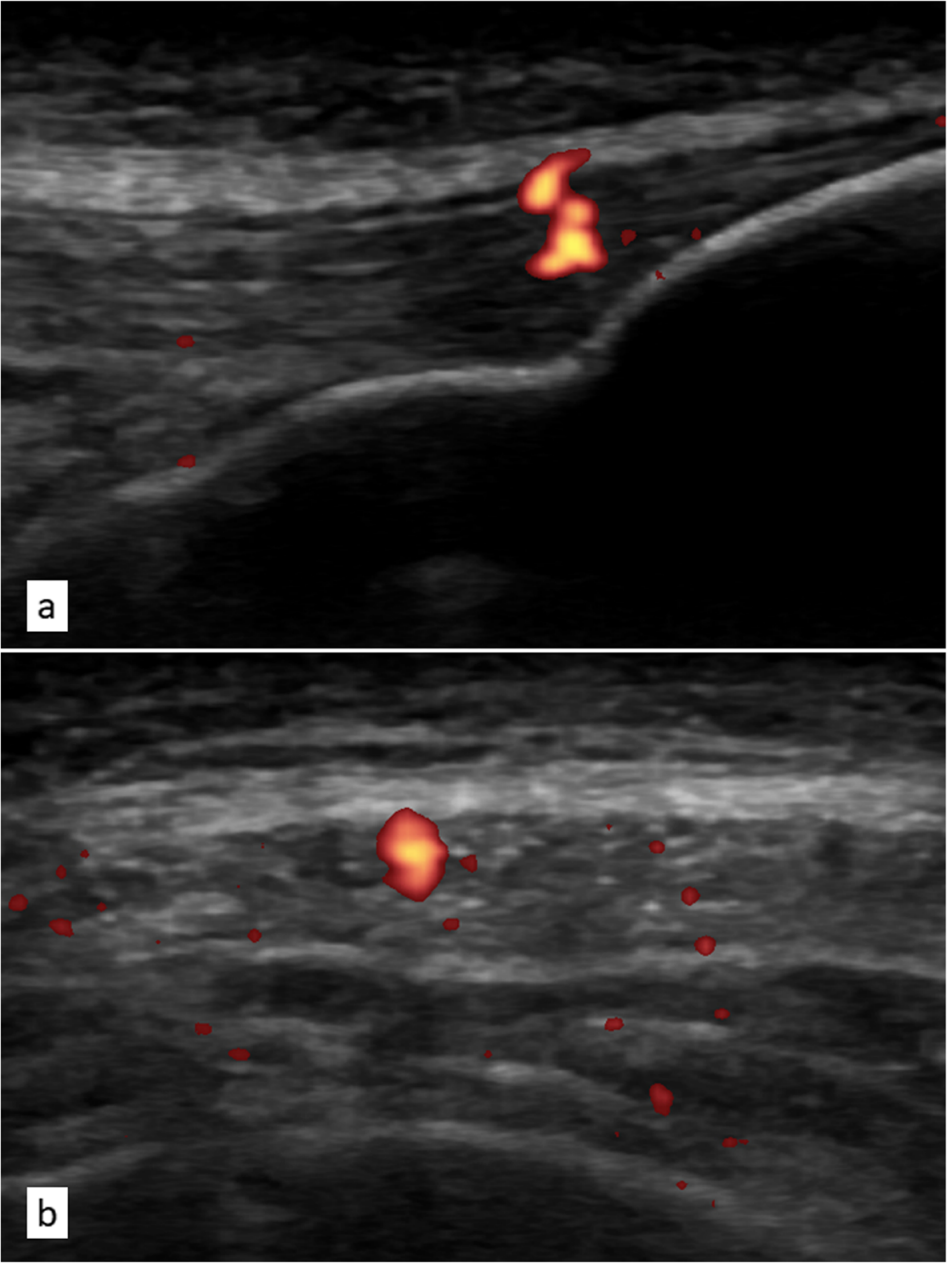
Orthogonal infrapatellar images of the distal patellar tendon with positive Doppler findings. Legend: a. Longitudinal image. b. Transverse image
